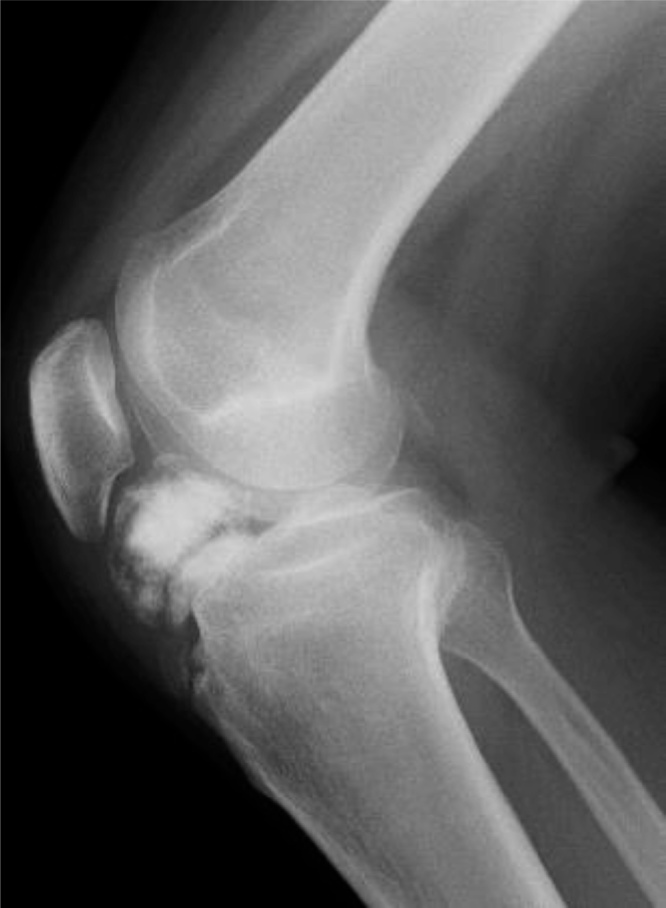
Plain radiography (lateral view) showed a finely circumscribed osseous lesion in the infrapatellar fat pad.
